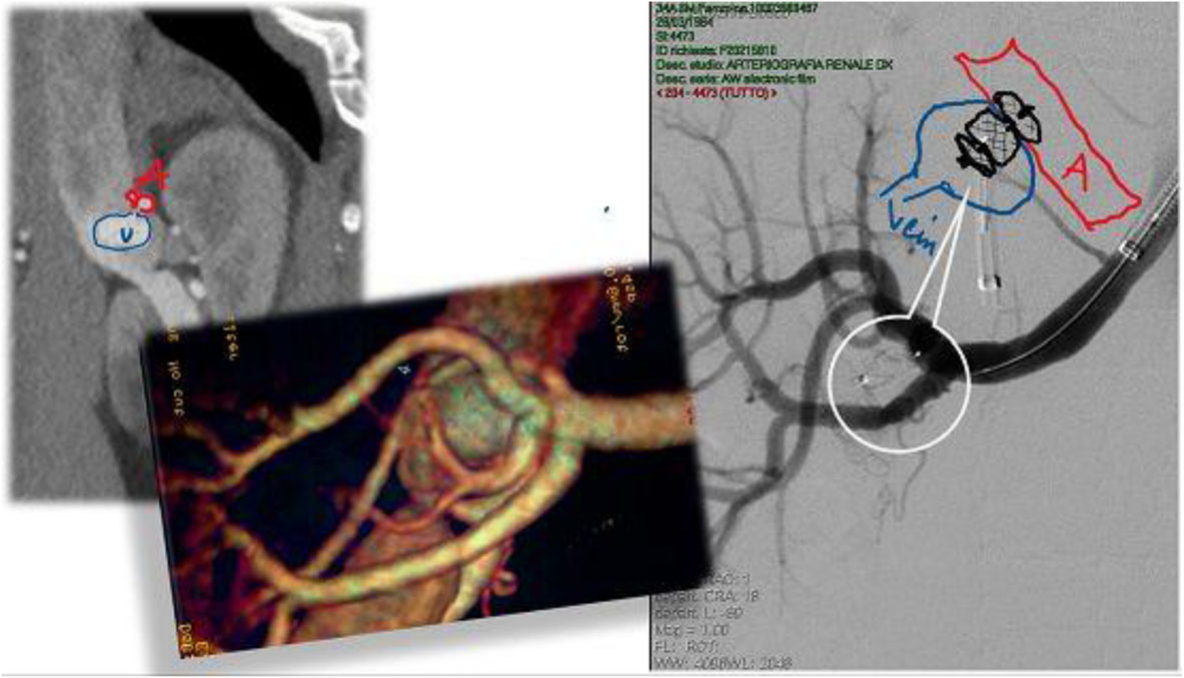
Left: CT and VR view of the communication point of the CRAVF. Right: Amplatzer Vascular Plug II released at extact level of the arteriovenous comunication
